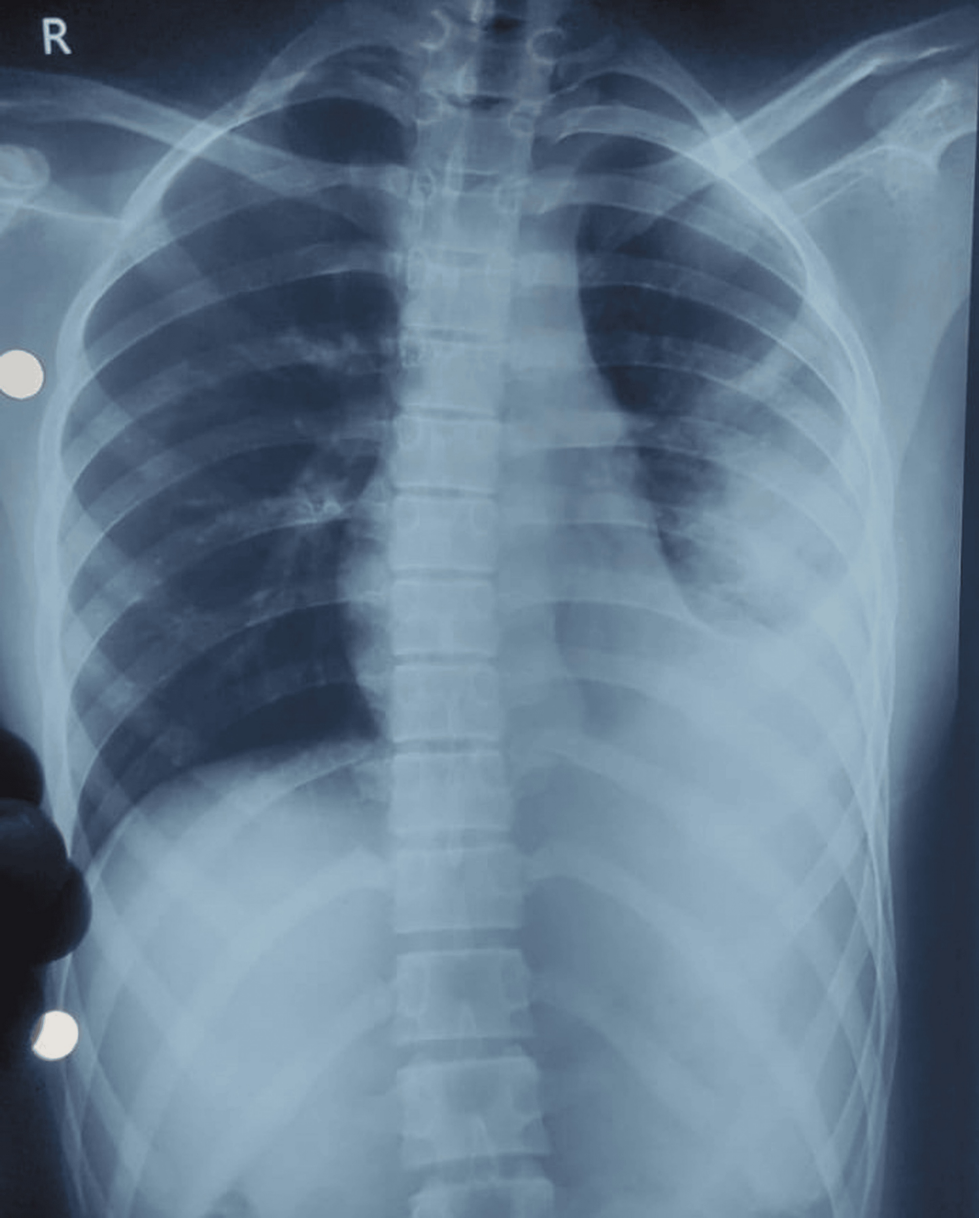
Chest X-ray showing left sided pleural effusion (post-thoracocentesis)
